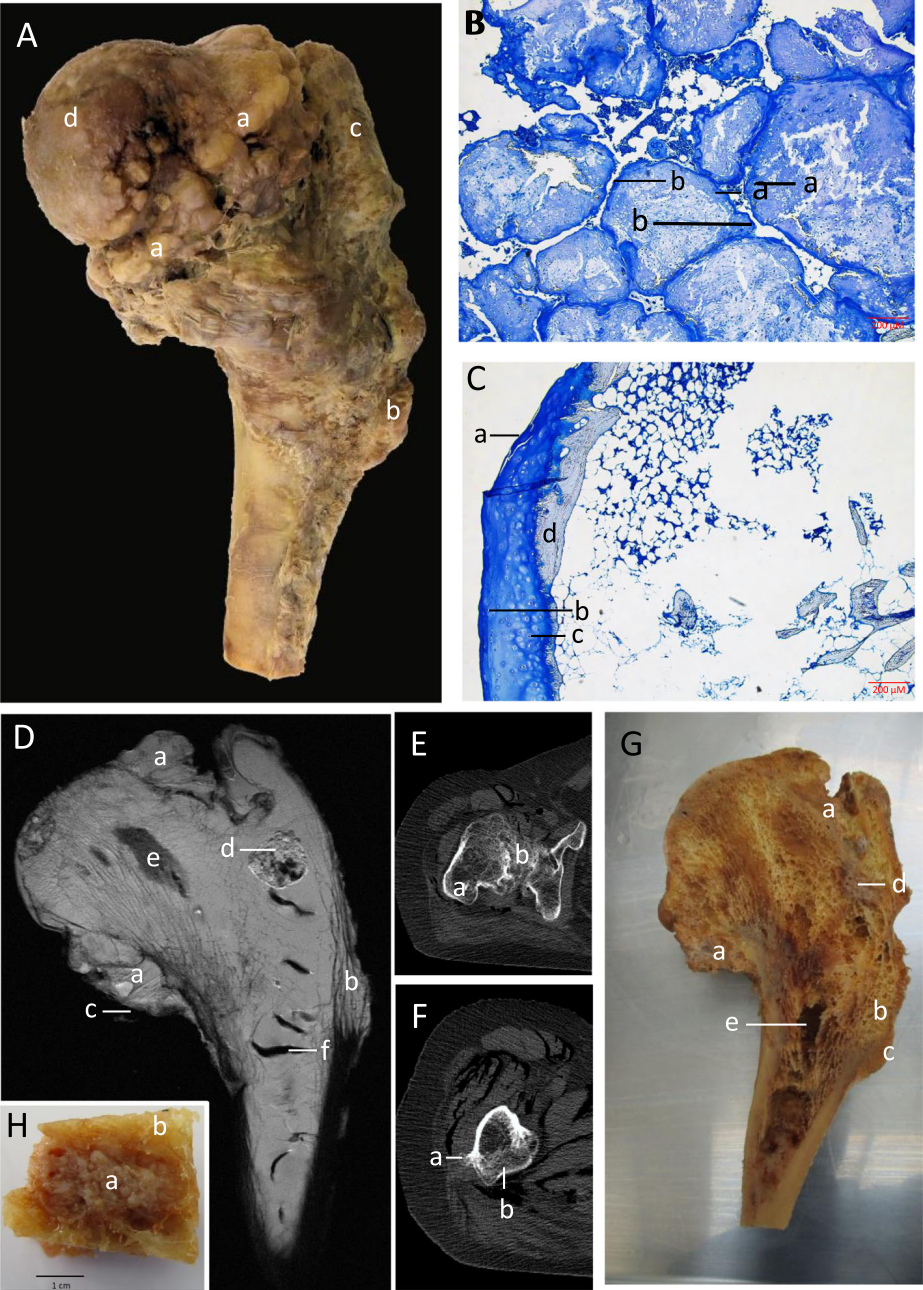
Right proximal femur. a Posterior view. A large exostosis (a) surrounding the metaphysis with an outgrowth (b) from the lateral portion of the femoral shaft. Greater trochanter (c) and femoral head also displayed (d). b A section through intramedullary mass. (a) Cartilage matrix (b) inter nodular cartilage. c A section through the surface of a sessile osteochondroma. (a) Perichondrium on surface of cartilage cap. (b) chondrocytes. (c) Hypertrophic mature chondrocytes. (d) Subchondral bone. d T2 MRI coronal section. Osteochondromas can be seen surrounding the metaphysis and extending from the diaphysis (a and b respectively). Cartilage caps can also be seen on the osteochondromas (c). A large fluid filled mass is present in the metaphysis (d). Large voids are present in both the epiphysis and diaphysis (e and f). e Transverse CT scan through greater trochanter (a). Showing osteochondroma (b). f Transverse CT scan through proximal diaphysis. The pedunculated osteochondroma (a) can be seen on the lateral surface whilst the sessile growth can be seen on the posterior diaphysis (b). g Coronal section. (a) Cross section of osteochondroma. (b) trabecular bone obliterating normal cortical bone as the medulla of the overlying exostosis. (c) Cortex of exostosis. (d) Soft mass located in the metaphysis. (e) An example of the gaps located within the trabecular bone. h Cartilaginous mass removed (a) surrounded by trabeculae (b)
